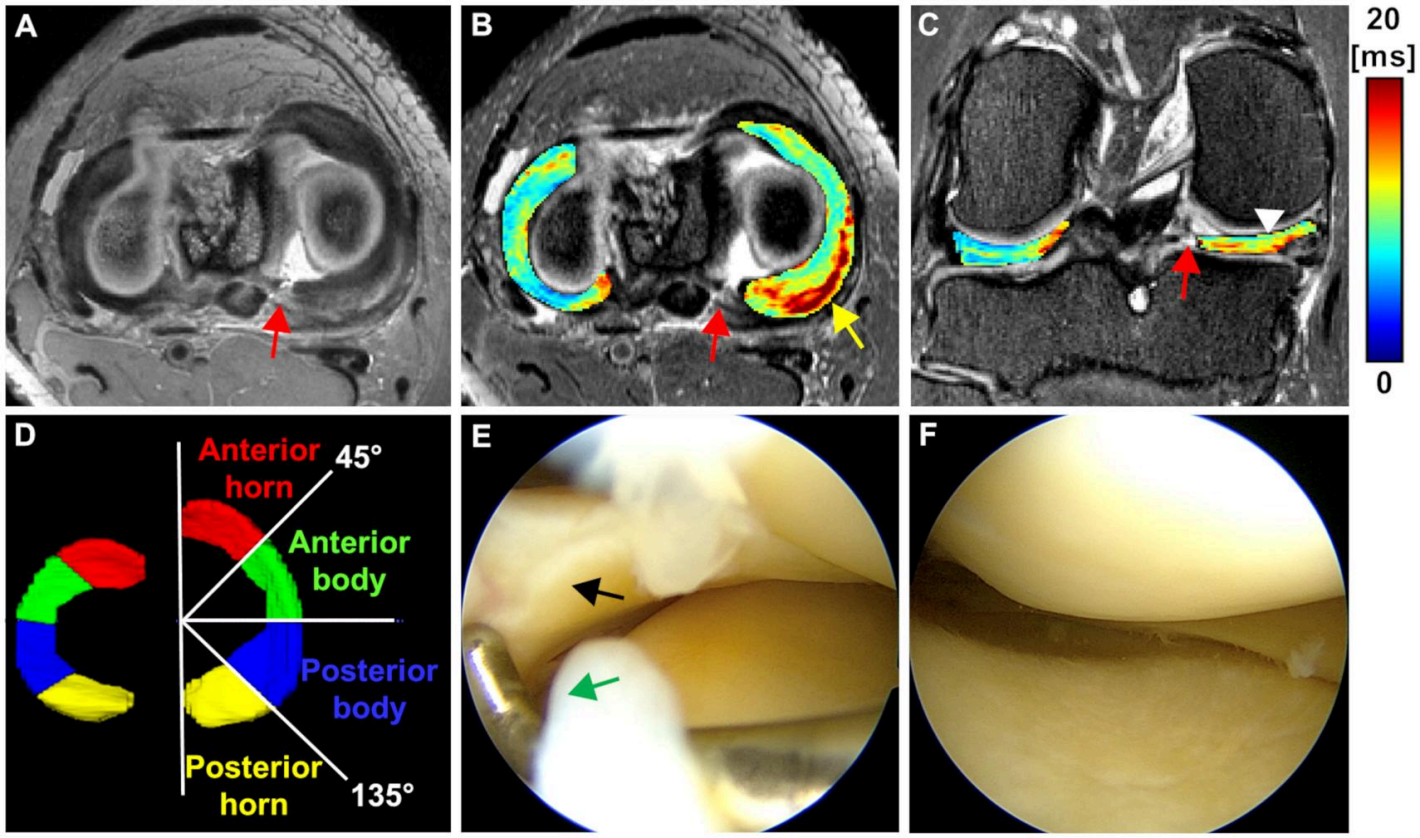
Representative MRI and arthroscopy images of a patient (age 57 years, female) with a posterior horn tear in the medial meniscus. A) Axial SPACE PD weighted image with arrow pointing to the root tear. B) Axial T2* map of the lateral and medial menisci overlaid on the axial SPACE T2 with fat suppression. Elevated T2* values are depicted in the posterior region proximal to the root tear (arrow pointing to the colormap). C) Coronal T2* map of the lateral and medial (arrowhead) menisci overlaid on the coronal SPACE T2 with fat suppression. D) 3D regional segmentation of the lateral and medial (regions annotated) menisci for quantitative analysis. E-F) preoperative arthroscopic images show the smooth surface of the medial meniscus. The arrows point to the medial meniscus root and the root attachment. The colour bar depicts T2* relaxation in milliseconds.
